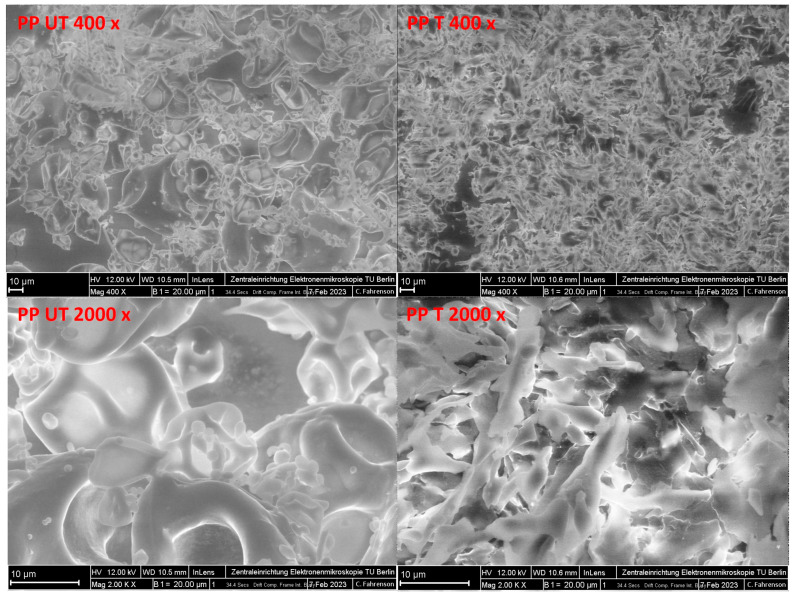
FE-SEM images (400× and 2000× magnifications, scale bar: 10 µm) of untreated (UT) and ultrasound-treated (T) pea protein samples (PP).
